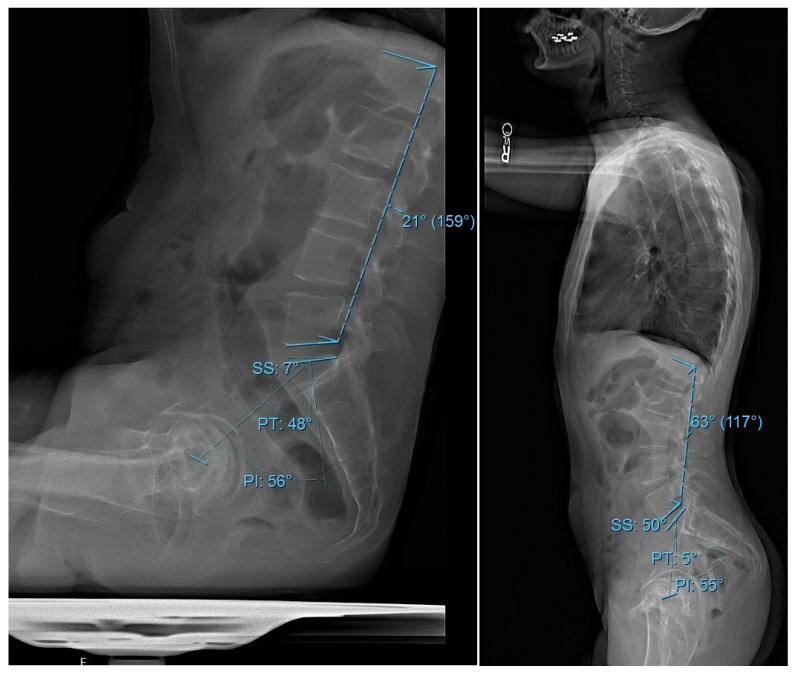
Spinopelvic measurements in sitting and standing XRs. Lateral radiographs of an idealized patient demonstrating the measurements of spinopelvic parameters. The parameters include pelvic incidence (PI), pelvic tilt (PT), sacral slope (SS), and lumbar lordosis (LL): on the left panel in sitting and on the right panel in standing.
